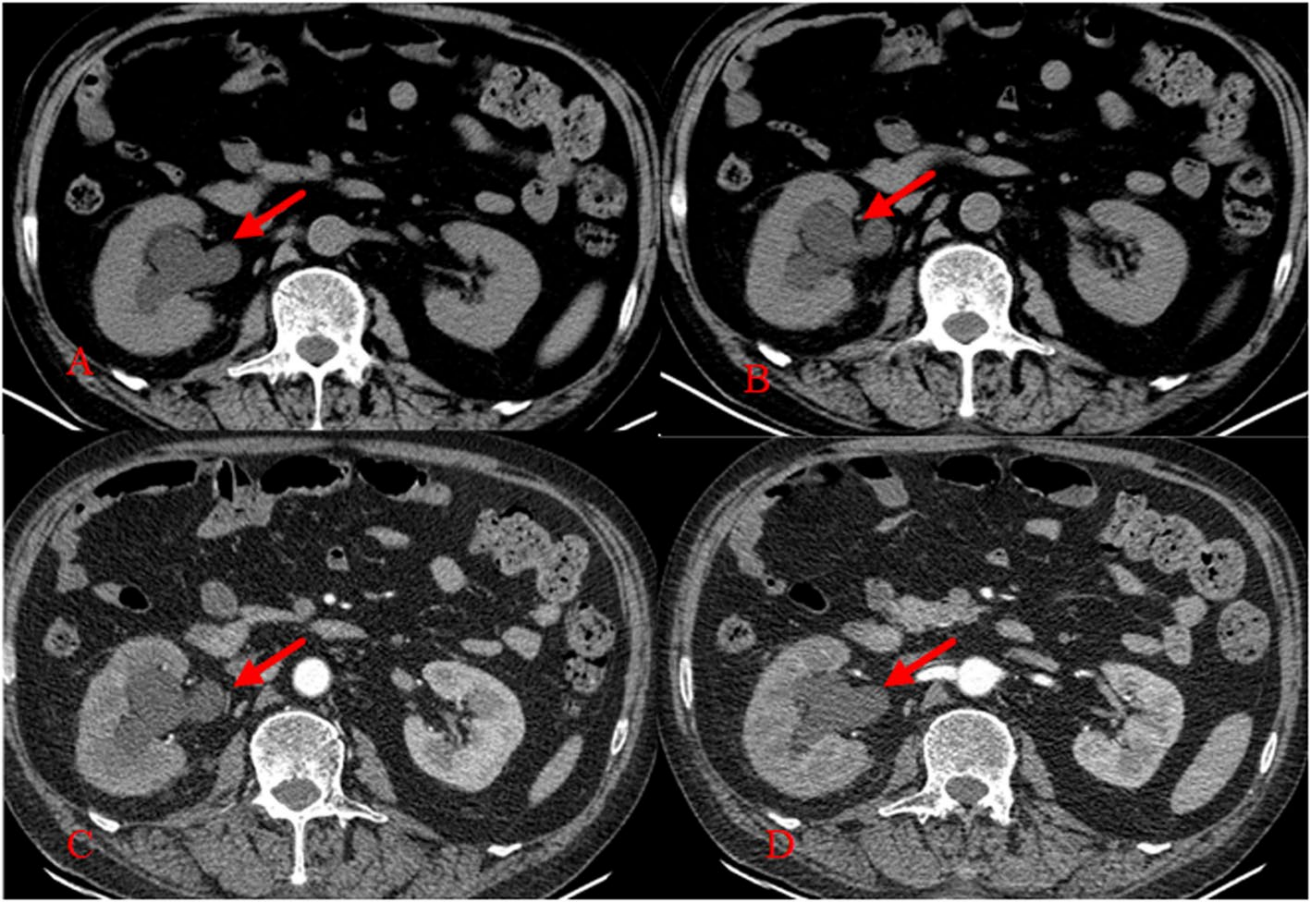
Contrast-enhanced CT scan of abdomen (2020–08-18). space-occupying lesions was located at the lower part of the ureter (about 4.8*5.7 cm in size), which was not clearly separated from the adjacent right ureter and the right psoas major muscle (A-D)
